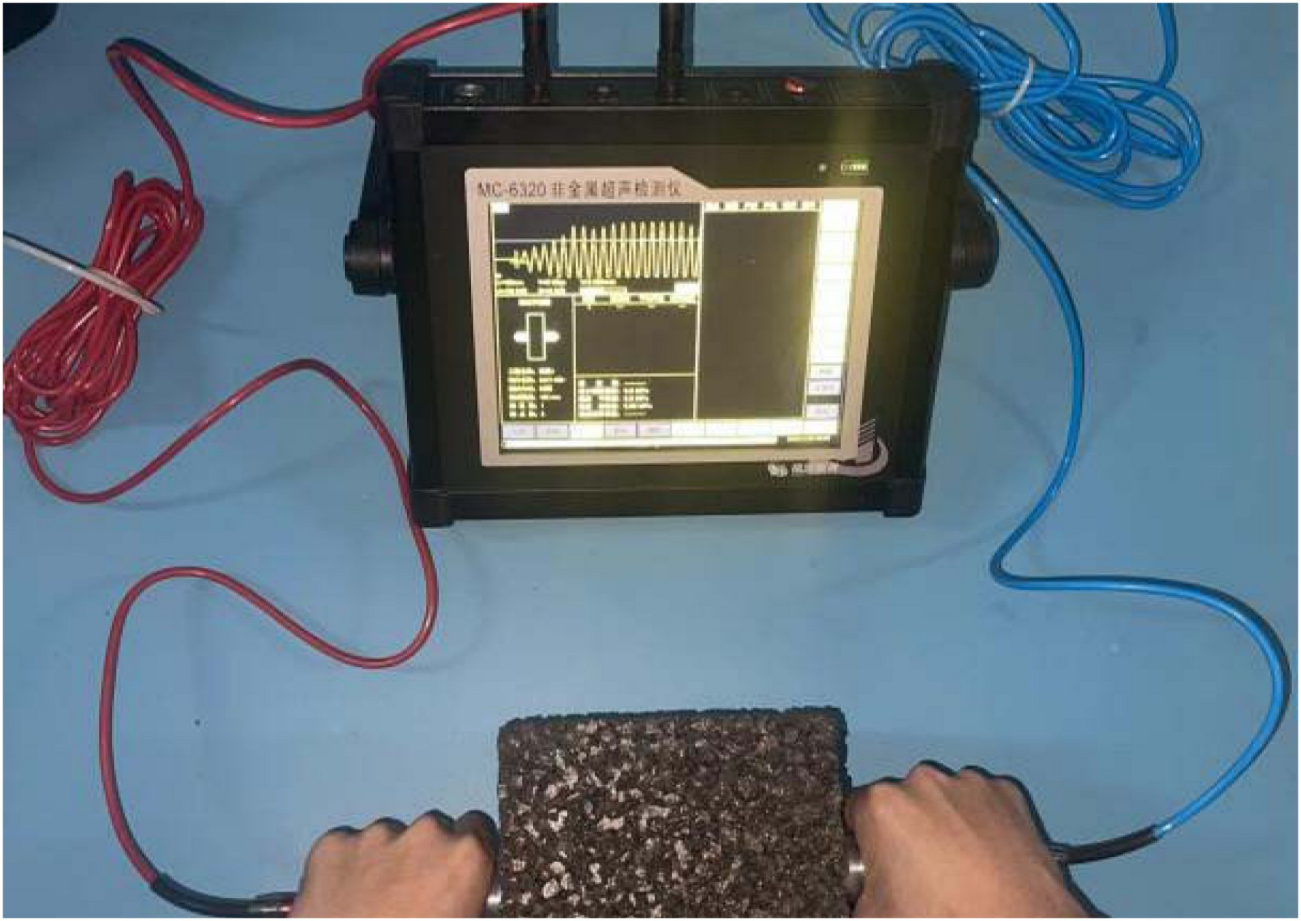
Ultrasonic velocity acquisition by MC-6320 non-metal ultrasonic detector.
